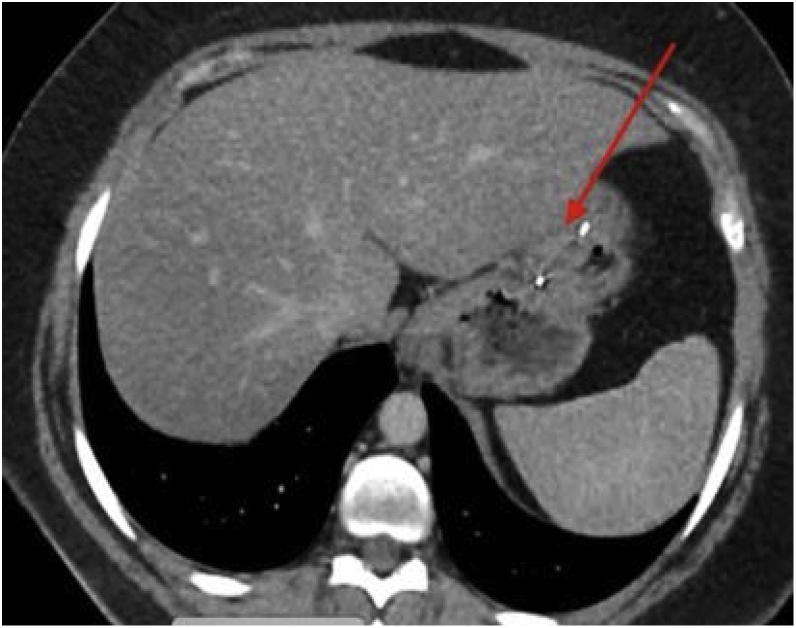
CT Axial image of deformed stomach with ESG hardware.
